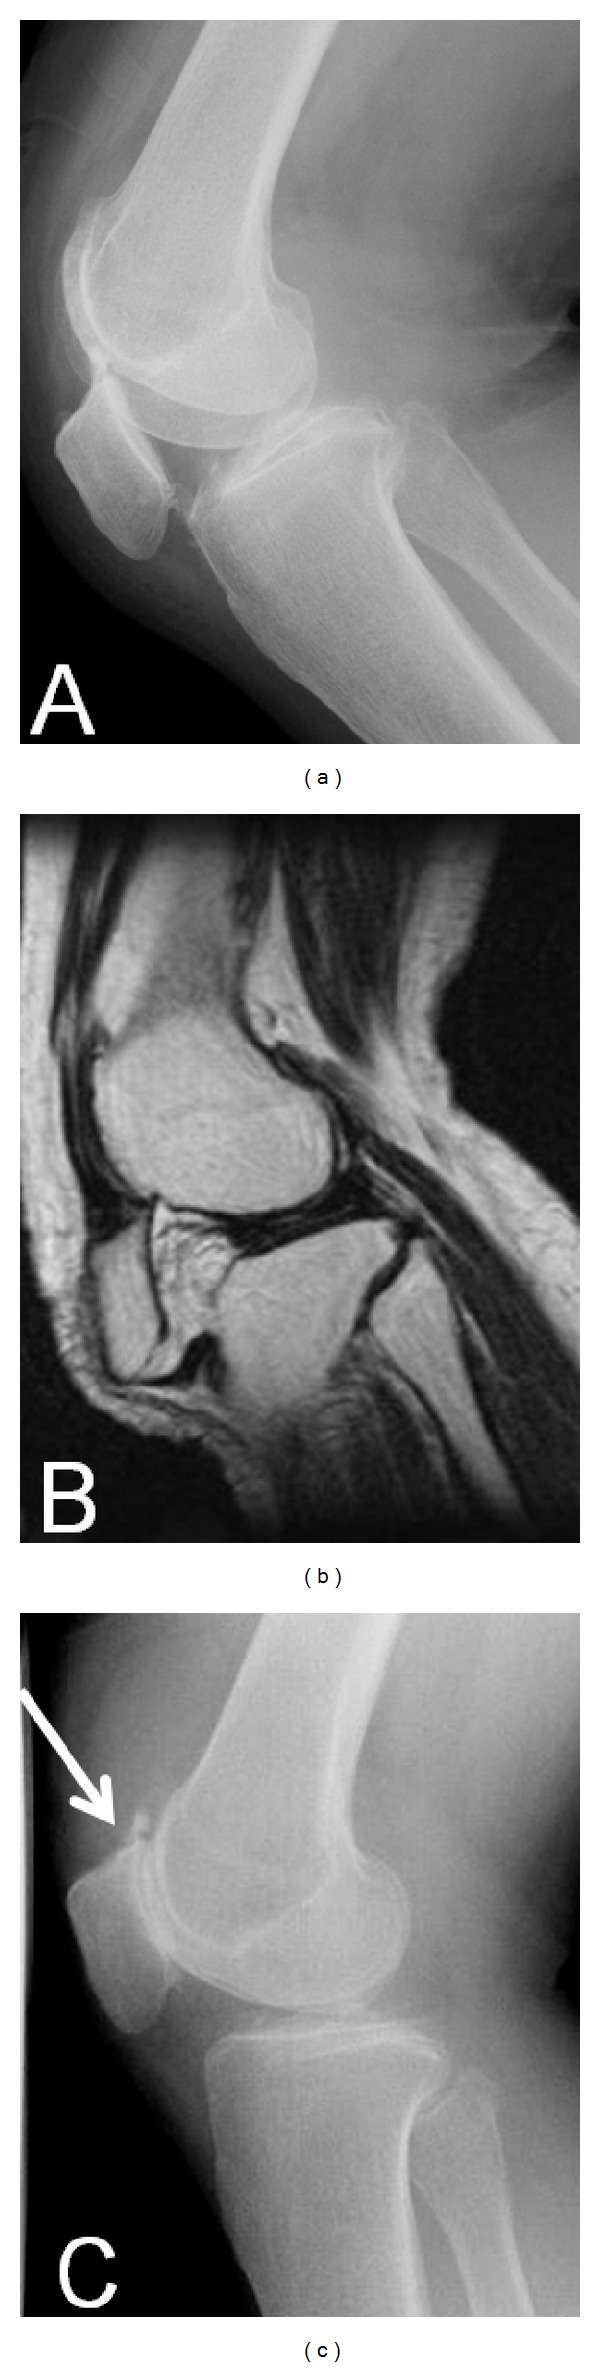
(a) Plain lateral radiography: the patella is dislocated inferiorly. (b) MRI: the osteophyte on the superior pole of the patella has become impacted in the femoral trochlea. (c) Plain radiography after manual reduction: the osteophyte on the superior pole of the patella is completely fractured (arrow).
